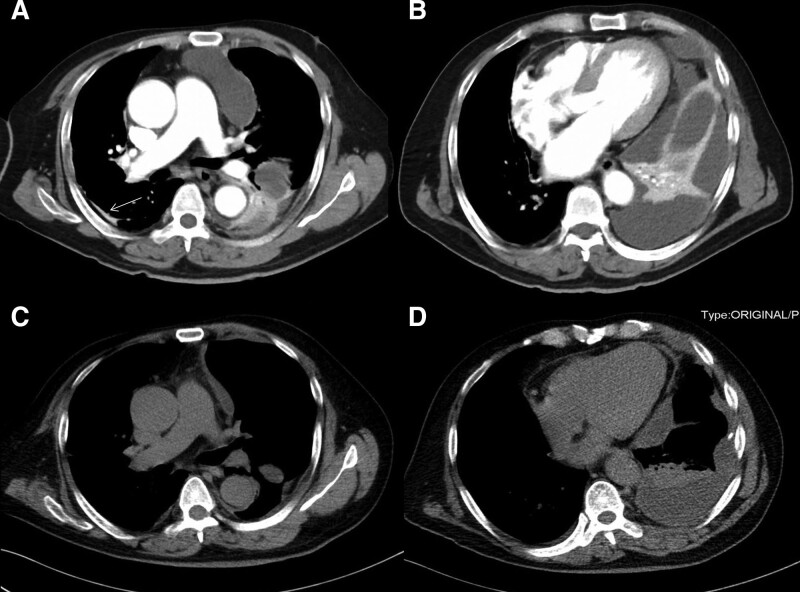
Image of Thoracic CT. (A and B) Thoracic CT scan on admission. The image showed a massive pleural effusion on the left side, which was wrapped locally, and local atelectasis in the right lung. (C and D) Thoracic CT scan on d 10 of hospitalization. The image showed that the left pleural effusion became smaller, with local encapsulation. CT = computed tomography.
